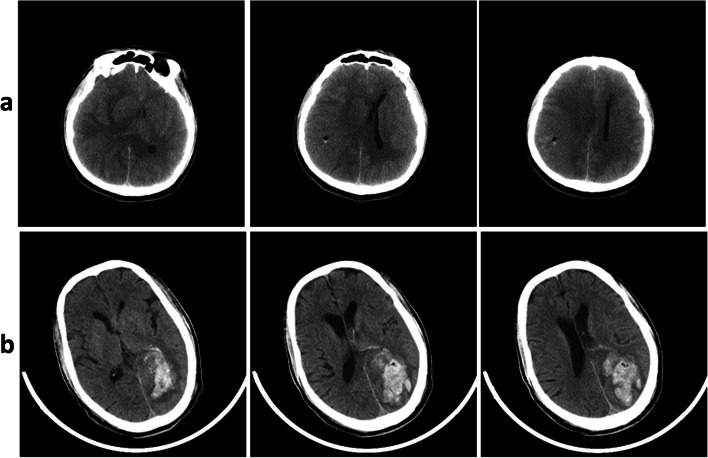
a High-density area measuring less than 5 mm in diameter at the biopsy puncture site on the postoperative CT scan was not considered to indicate hemorrhage. b Intraparenchymal hematoma measuring more than 5 mm in diameter in the postbiopsy CT scan image was positively defined as operation-related hemorrhage
